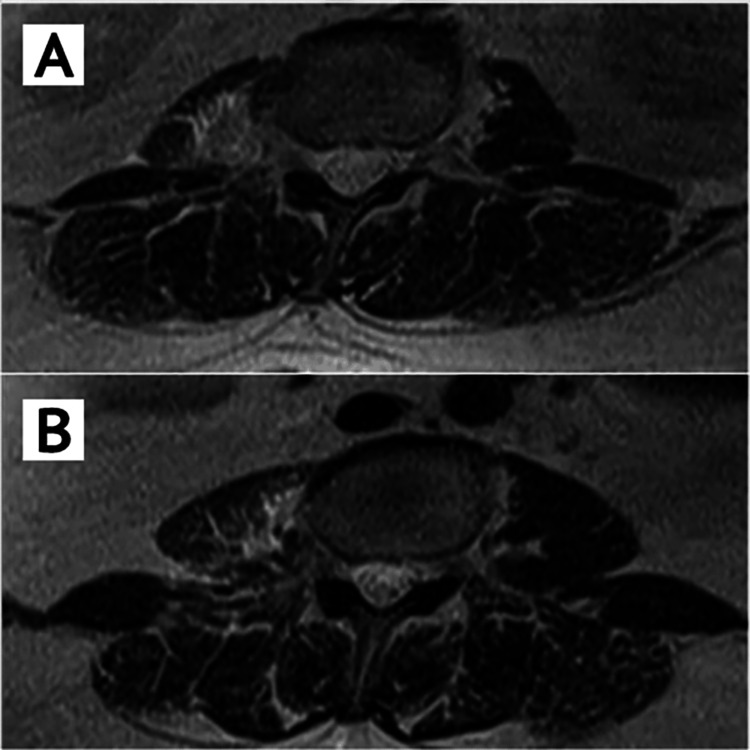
T2 axial MRI images (A) at L1-L2 level showing mild posterior disc herniation causing insignificant changes. No central canal stenosis or root compression (B) at the L2-L3 level was noted.
